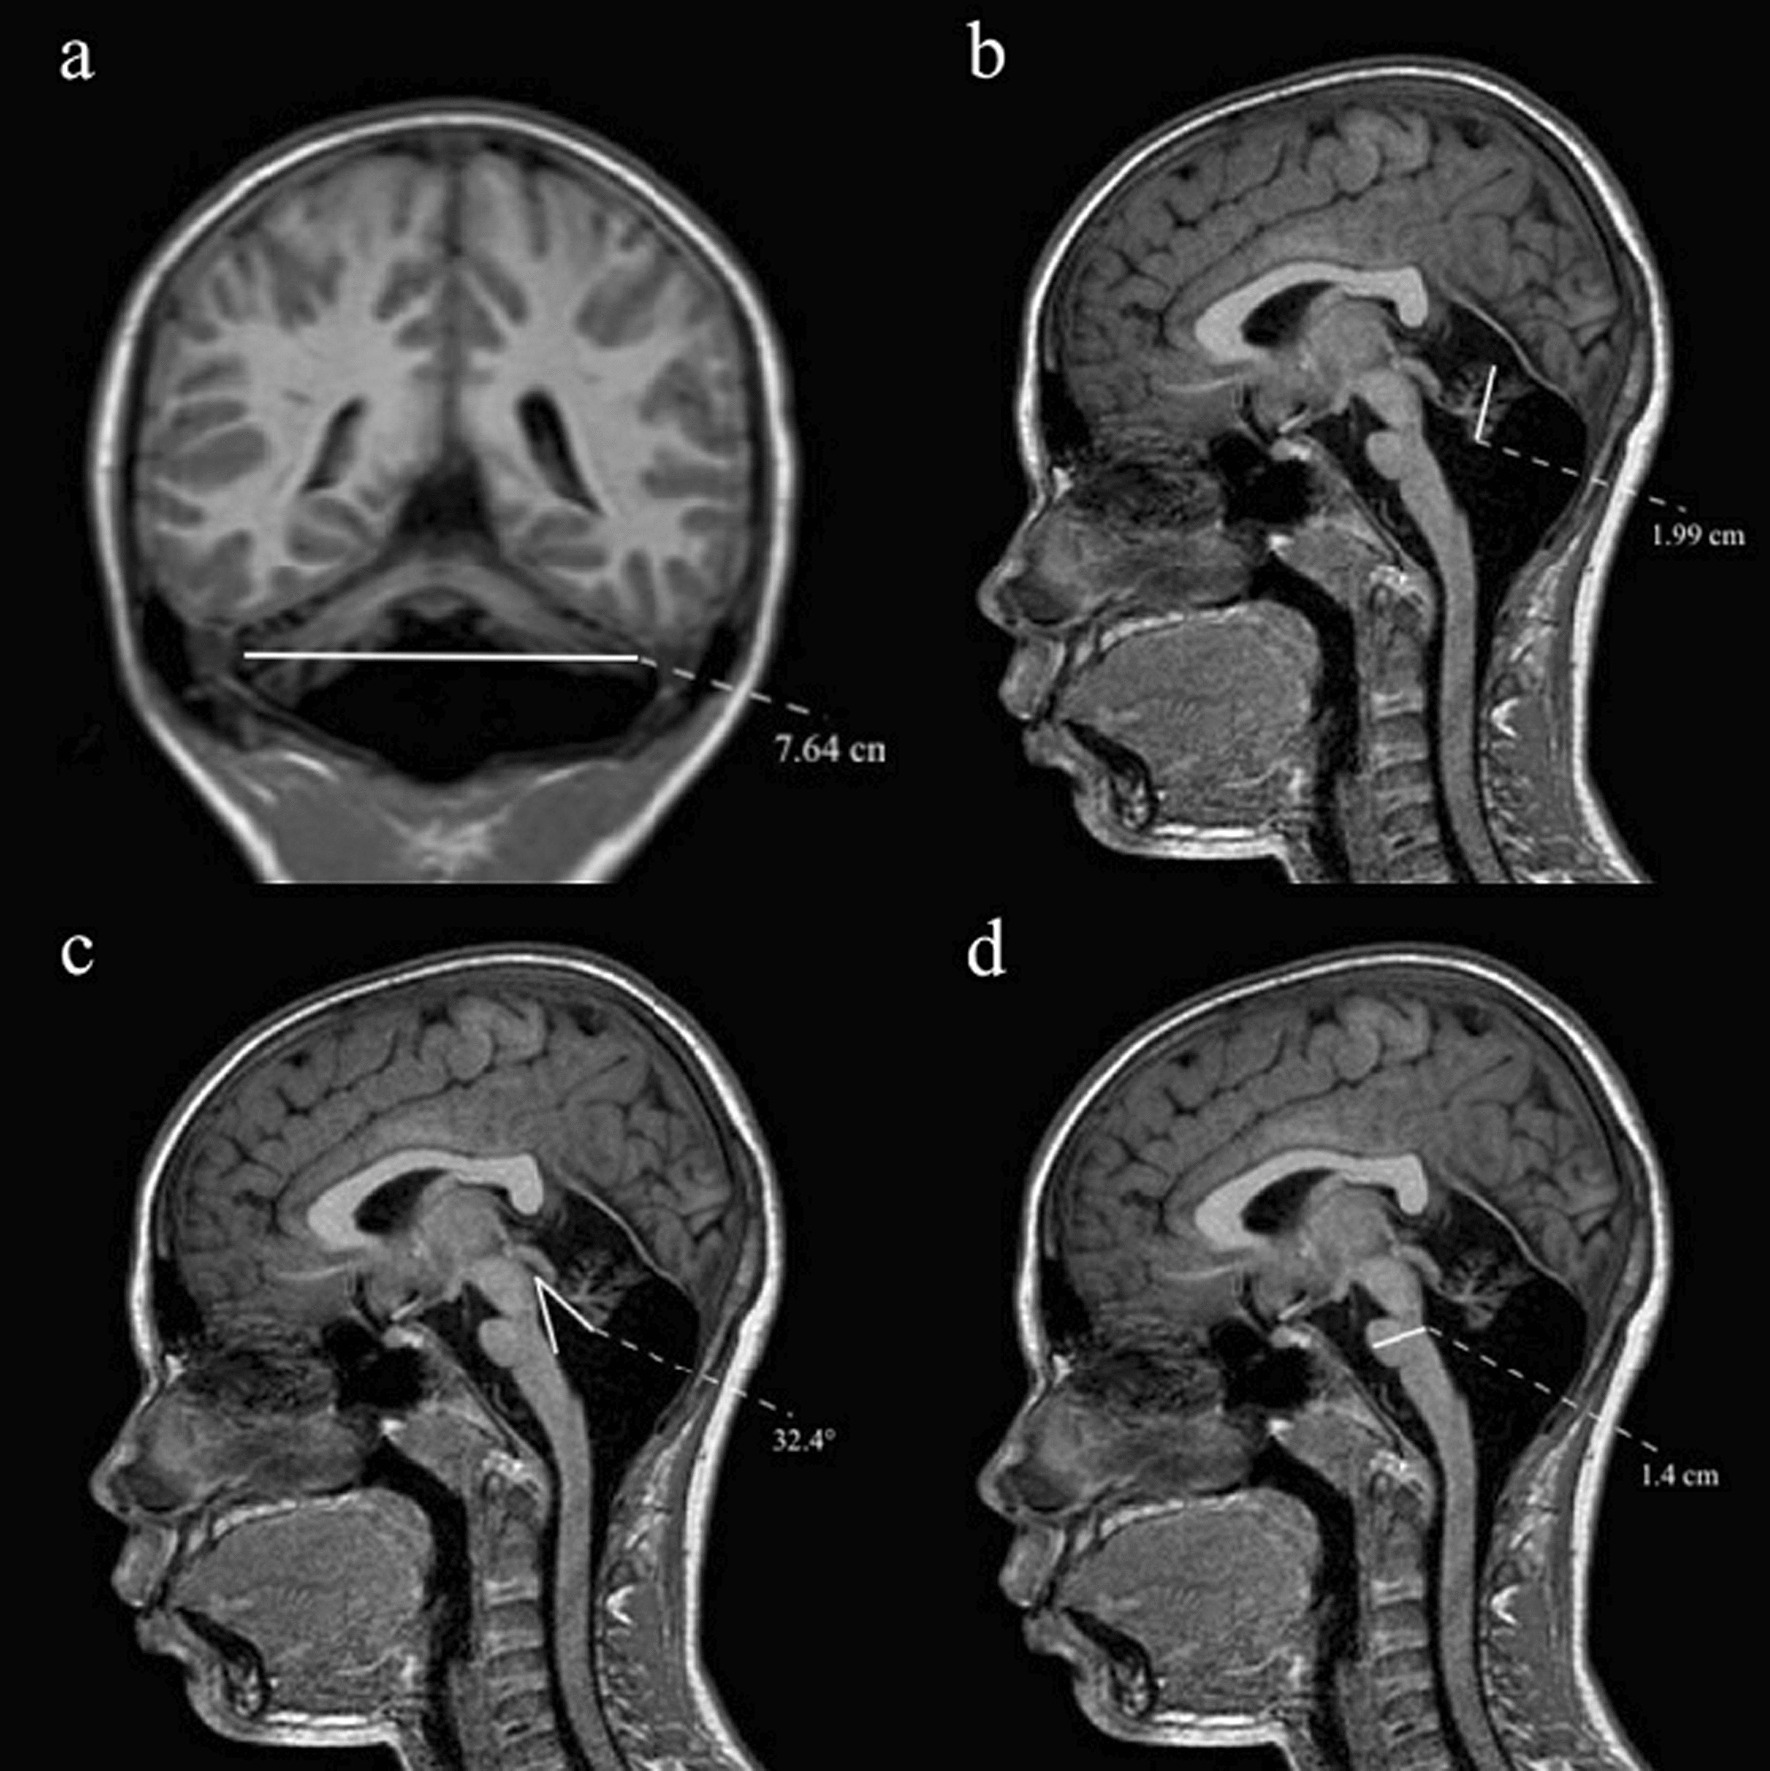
Representative brain MRI biometry (Patient 5). a Maximum transverse cerebellar diameter (MCD). b Maximum craniocaudal height of the vermis (CCD). c Tegmento-vermian angle (TVA). d Mid-pons diameter (a T1-weighted coronal image; b–d midline T1-weighted sagittal images)
